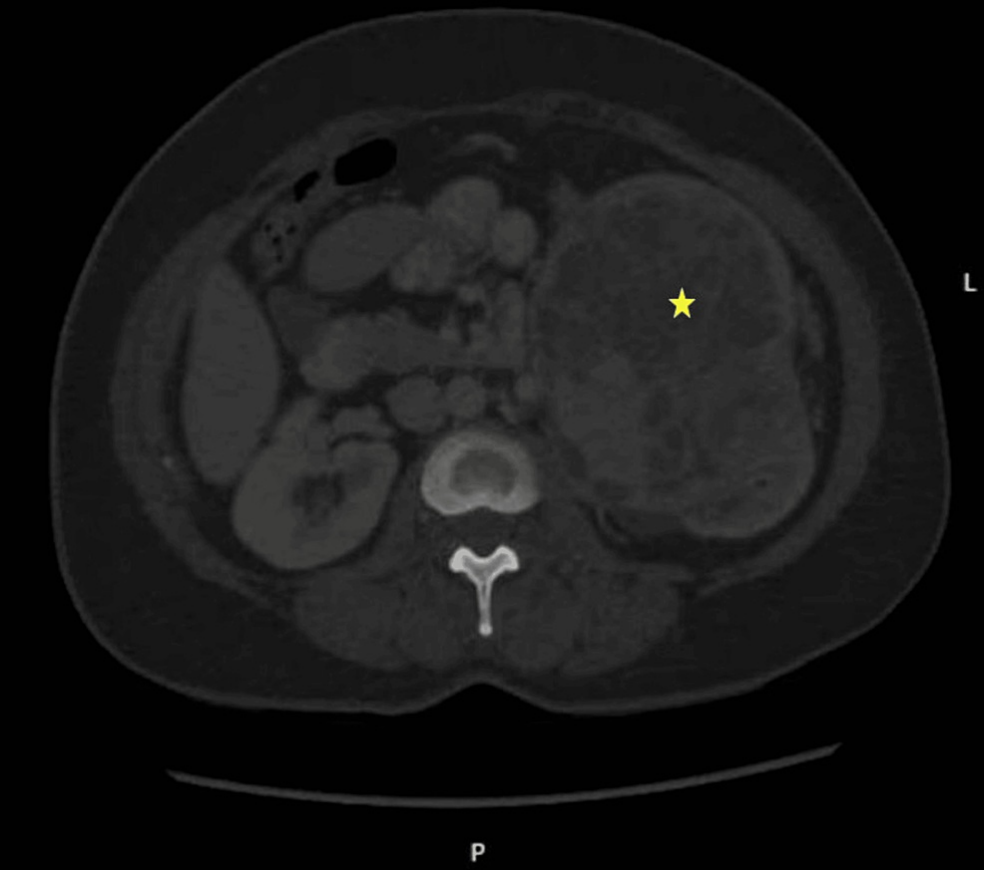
CT image Left kidney with a large intrarenal mass (yellow asterisk).
